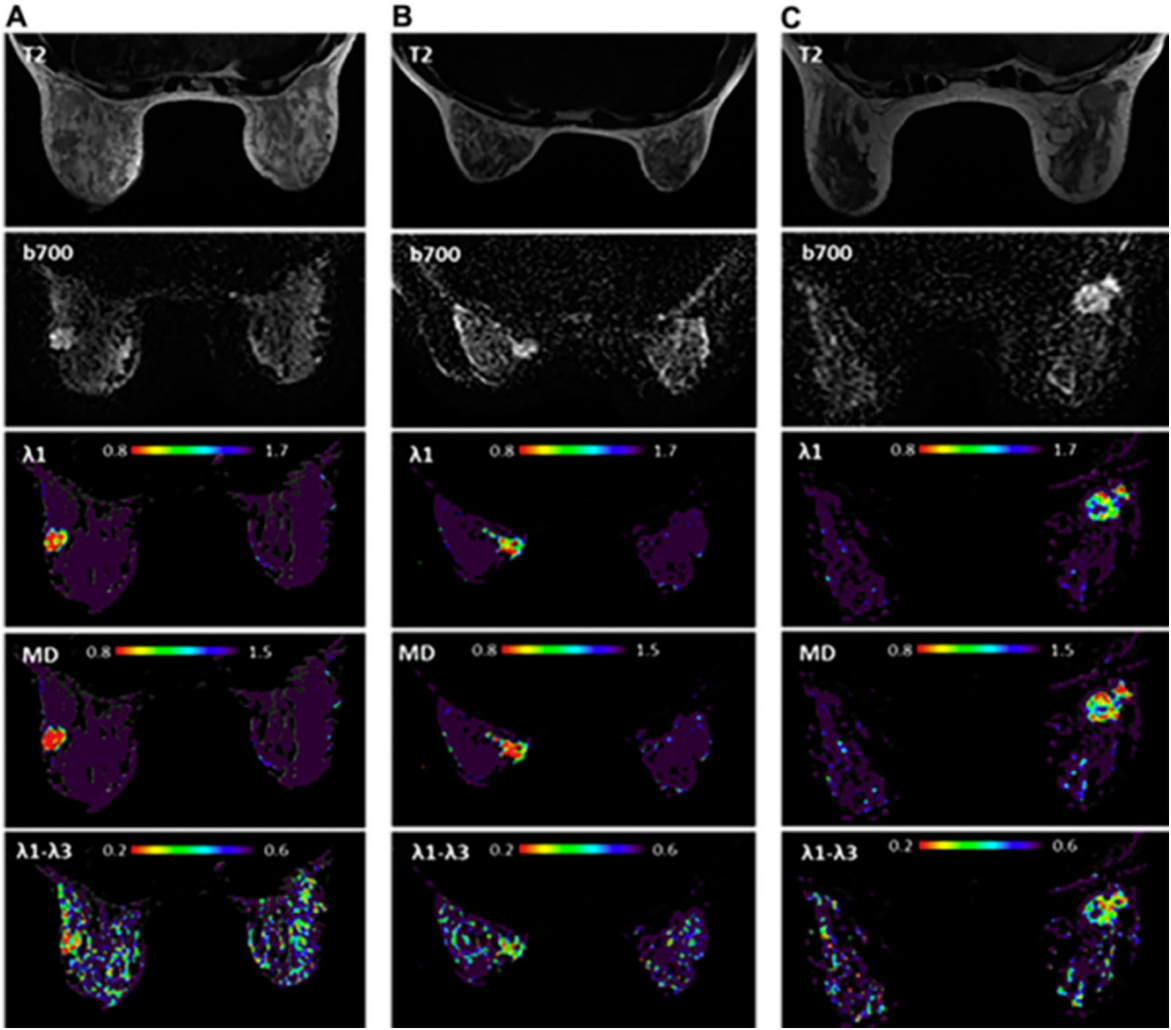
Unenhanced diffusion MRI during pregnancy. T2, DWI, and DTI parametric maps of three PABC patients (A–C). T2-weighted, DWI and DTI-derived diagnostic parametric maps of *λ*1, MD, and *λ*1–*λ*3 of three patients with newly diagnosed IDC are presented. The lesion appears bright on DWI (b 700 s/mm^2^). Using the parametric threshold, the lesion could be easily depicted on l1, and MD maps, as well as on l1–l3 map, compared with the measurements in the normal tissue. Reproduced with permission from Journal of the American College of Radiology
